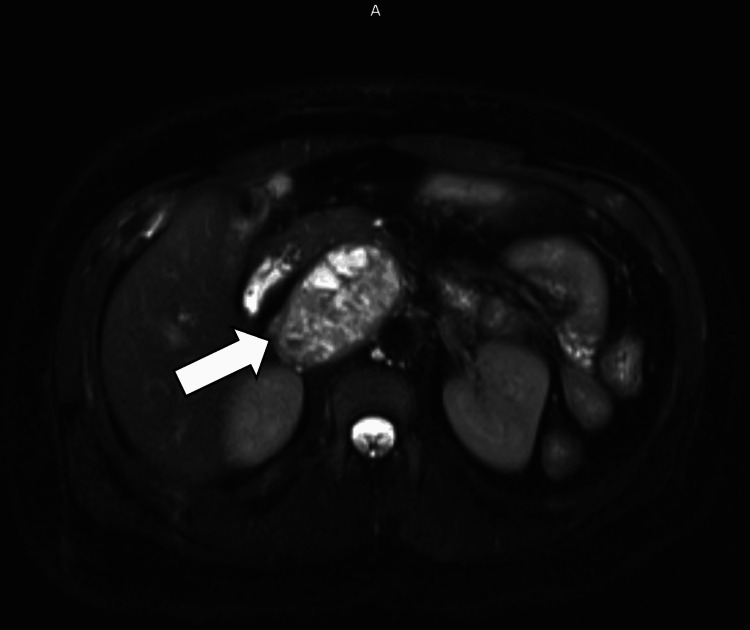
T2-HASTE (half-Fourier acquisition single-shot turbo spin-echo)-fast spin (fs) MRI abdomen showing a 6.4 cm right retroperitoneum mass
